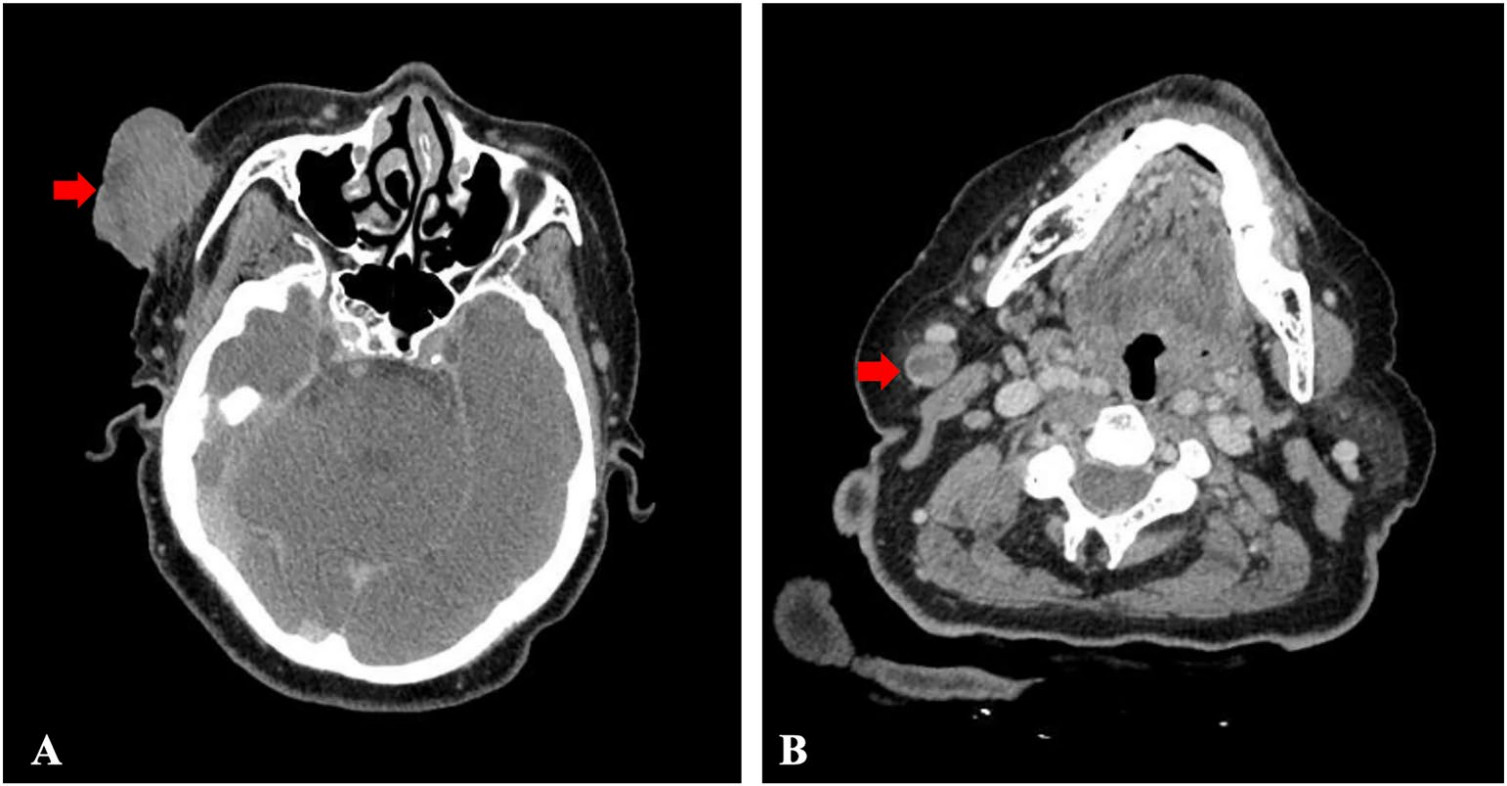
CT manifestations of right maxillofacial lesion and cervical lymphadenopathy: (A) An infiltrative soft tissue mass (arrow) in the right maxillofacial region demonstrating irregular margins and heterogeneous enhancement; (B) Right cervical lymph nodes exhibiting characteristic peripheral rim enhancement (arrowhead) with central hypodensity.
